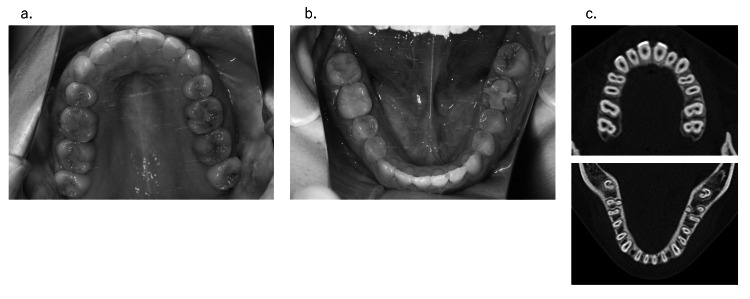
(a, b) Disharmony of the upper and lower arch forms. (c) CT imaging. The upper and lower widths will also not match if the maxillary molar bone is narrow or if the mandible is buccally expanded toward the posterior molar.
